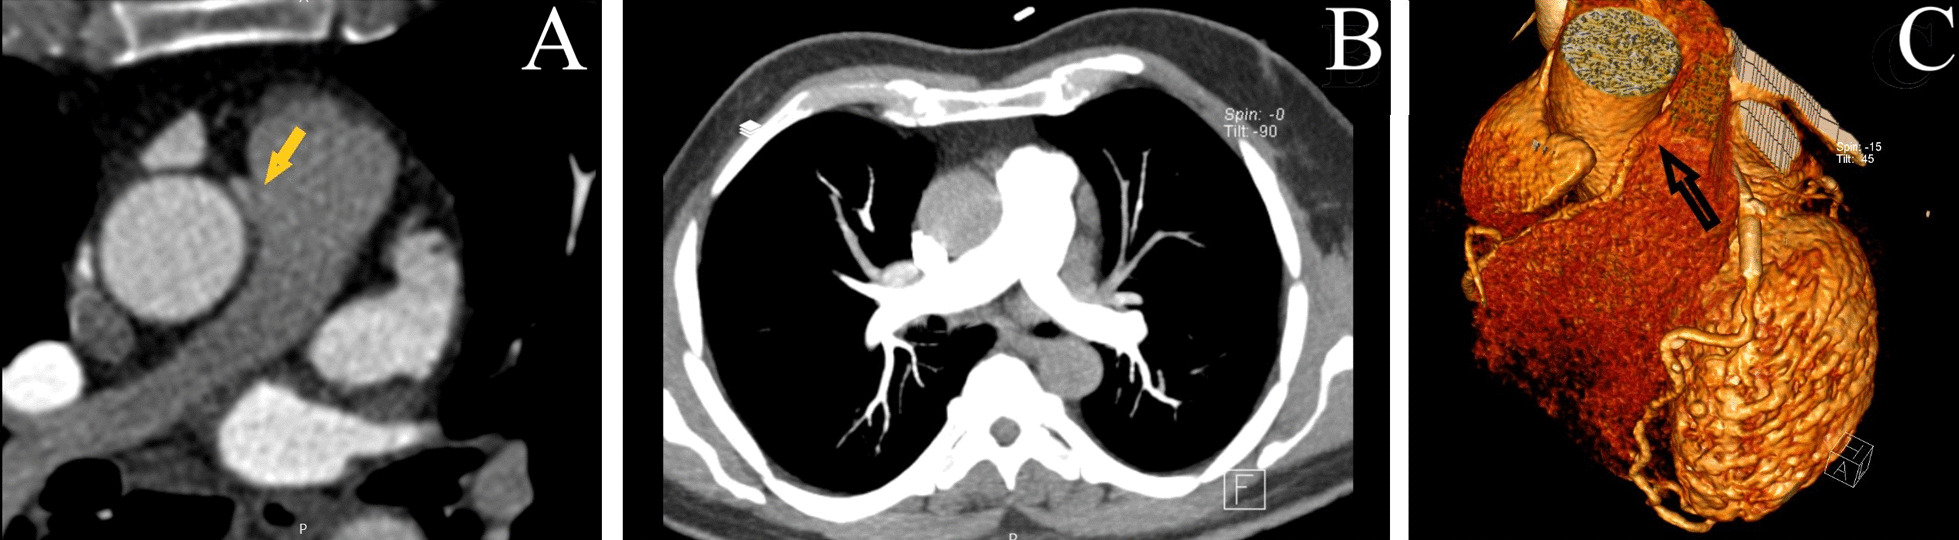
Computed Tomography Angiography. A Right coronary artery from the main pulmonary artery in the aortic phase. B No sign of right coronary artery in the pulmonary phase due to pressure gradient pre-operation. C Volume rendering technique (VRT), the origin of the right coronary artery from the main pulmonary artery
